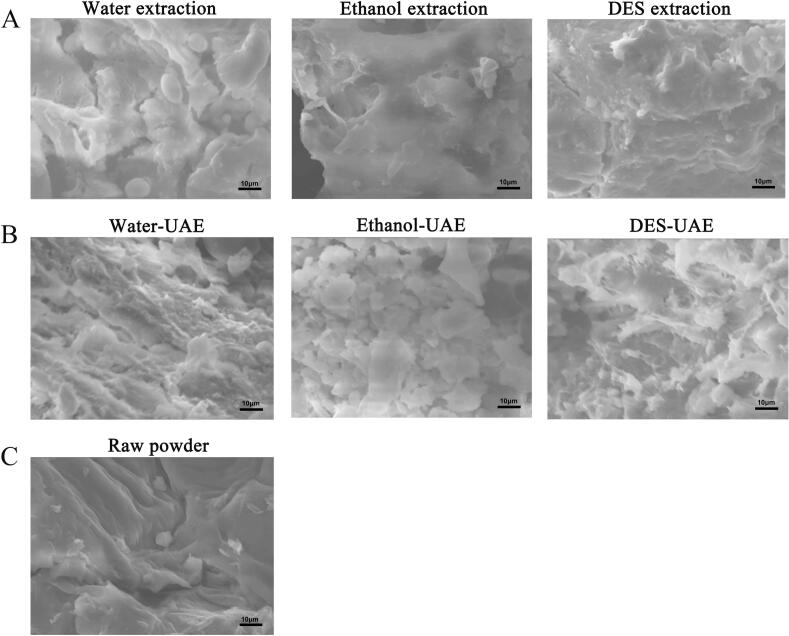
Morphological characterization of LSP with and without ultrasound treatment in different solvents. (A) Non-sonicated group, (B) Ultrasound-assisted extraction (UAE) group. (C) Raw LSP powder. All images were captured at 5000 × magnification; Scale bar: 10 μm.
